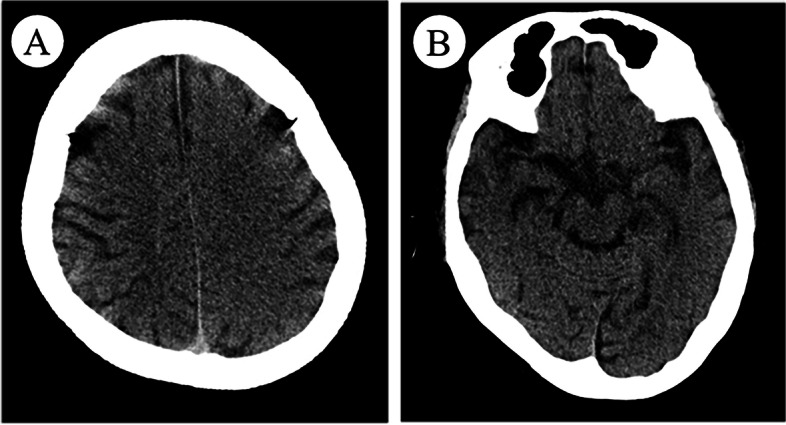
Repeat brain CT after twenty hours. Axial brain CT showed the resolution of left cerebral hemisphere swelling and diffuse high-density
